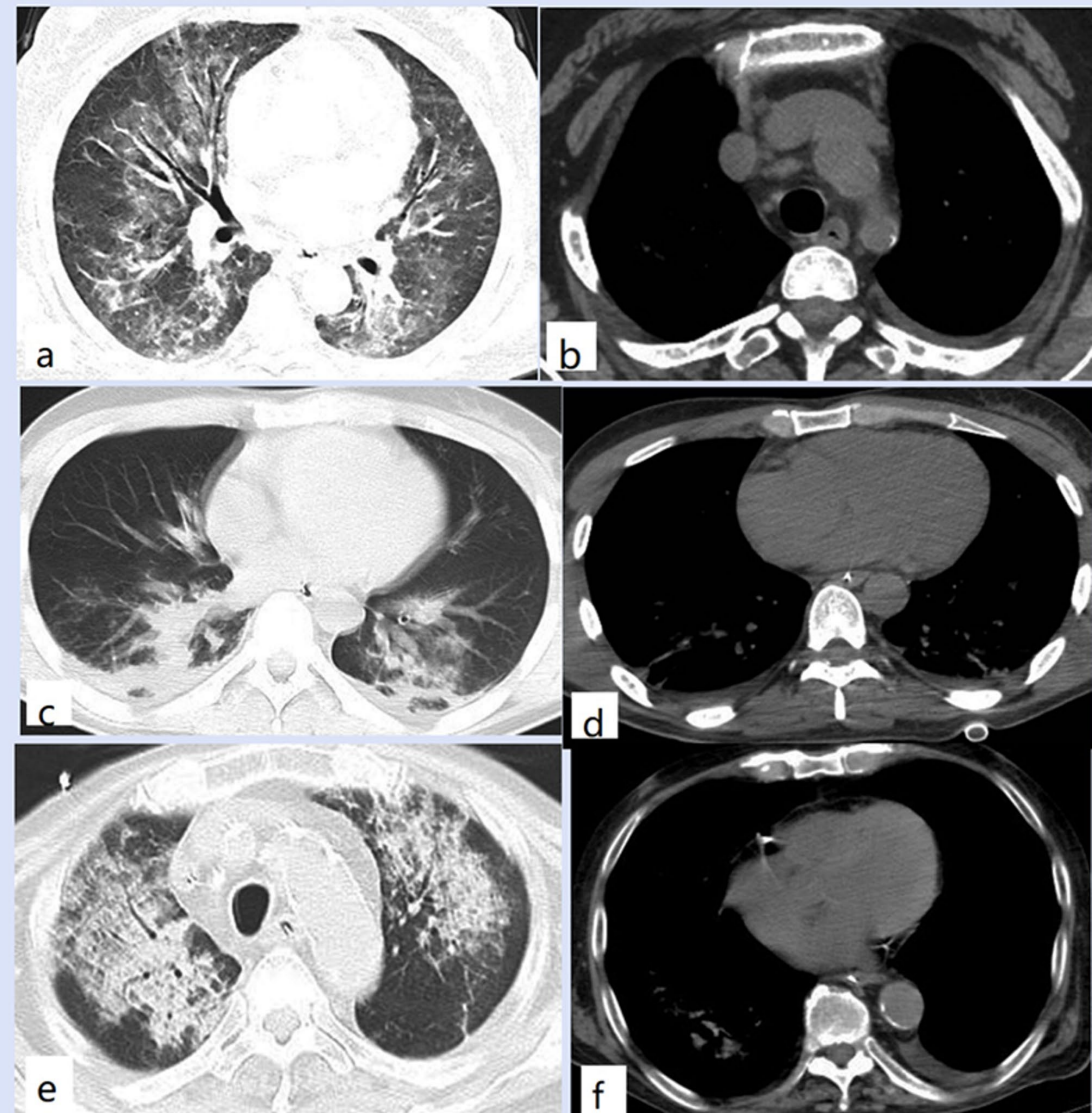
CT findings of pneumonia-type PC. Axial CT images showed bilateral ground-glass opacities with air-bronchogram (a), left pleural effusion, and mediastinal lymphadenopathy (b). Patchy consolidations could be seen in the bilateral lower lobes (c) and with bilateral pleural involvement and pleural effusions (d) on axial CT. Axial CT images showed diffuse consolidation-interstitial opacities in the bilateral upper lobes (e) with air-bronchogram (e) and left pleural effusion (f)
